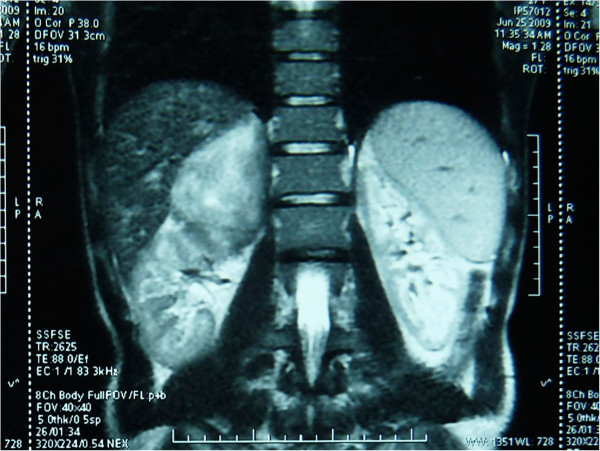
T2-weighted MRI: coronal section.
